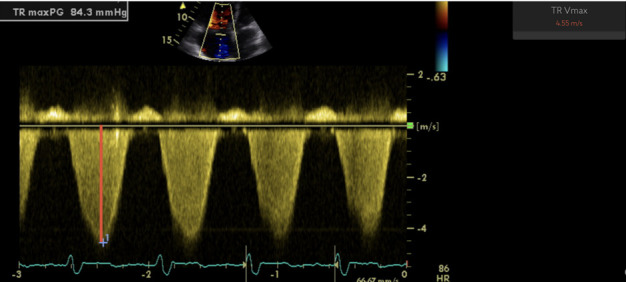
Echocardiographic measurement of peak tricuspid regurgitation jet velocity (TRJV). An echocardiogram showing a TRJV of 4.55 m·s^−1^ (red line), measured automatically, which corresponded to an estimated maximum tricuspid regurgitation pressure gradient of 84.3 mmHg, indicative of severe pulmonary hypertension (PH).
